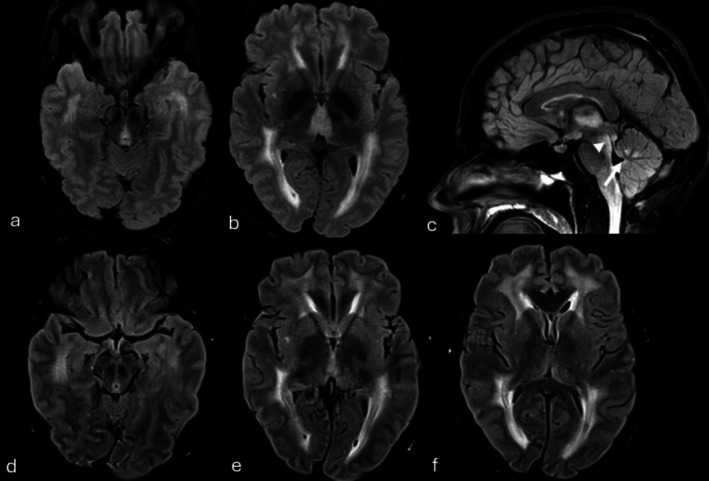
Wernicke's encephalopathy. Axial (a, b) and midsagittal (c) FLAIR images show bilateral symmetrical hyperintensity in the medial thalamus, mammillary bodies, periaqueductal gray matter and inferior colliculi (c, arrowhead), cerebellar vermis (c, white arrow), consistent with Wernicke encephalopathy. A one‐month follow up MRI shows the pre‐existent severe supratentorial leukoencephalopathy while the Wernicke's encephalopathy related changes had disappeared (d–f).
